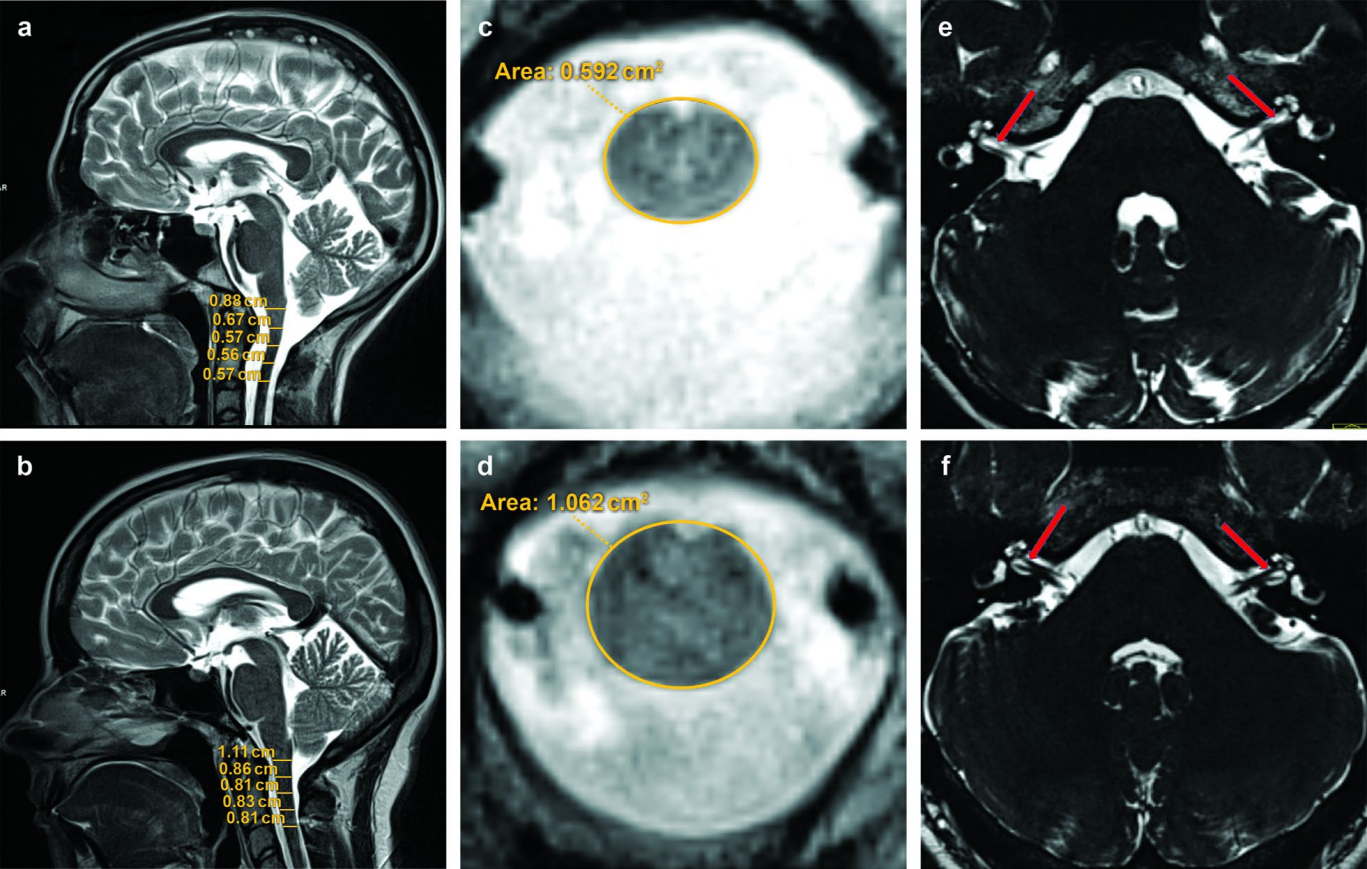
Brain and spine MRI in the proband. The *left column* shows sagittal T2 weighted images of the head and cervical spine (a proband, b control) with the measurements of spinal cord thickness at different levels. The *middle column* shows cross-section of the spinal cord (c proband, d control). The *right column* shows transverse heavy T2 weighted images of sub-millimeter slice thickness displaying the cochlear nerve, pointed with a* red arrow* (e proband, f control)
